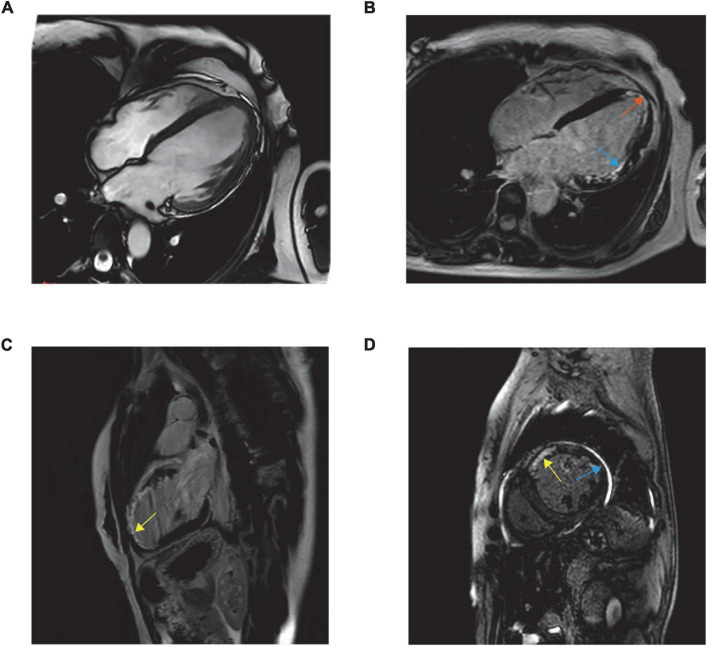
Balanced steady-state free precession MR images are obtained in standard cardiac planes. Long-axis and four-chamber view (A), long-axis and four-chamber late gadolinium-enhanced MR image (B), long-axis and two-chamber view (C), and short-axis and two-chamber view (D).
